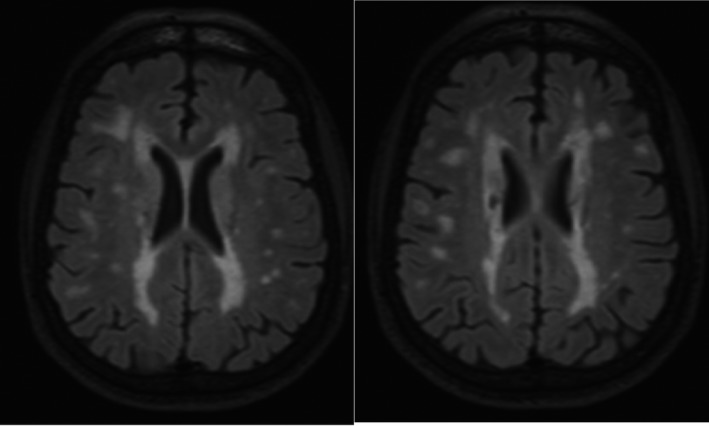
Axial planes of T2 FLAIR (fluid‐attenuated inversion recovery)‐weighted magnetic resonance imaging demonstrating multifocal subcortical and deep white matter hyperintensities involving both cerebral hemispheres, especially in the right frontal lobe, inferior parietal lobes, and bilateral external capsules
